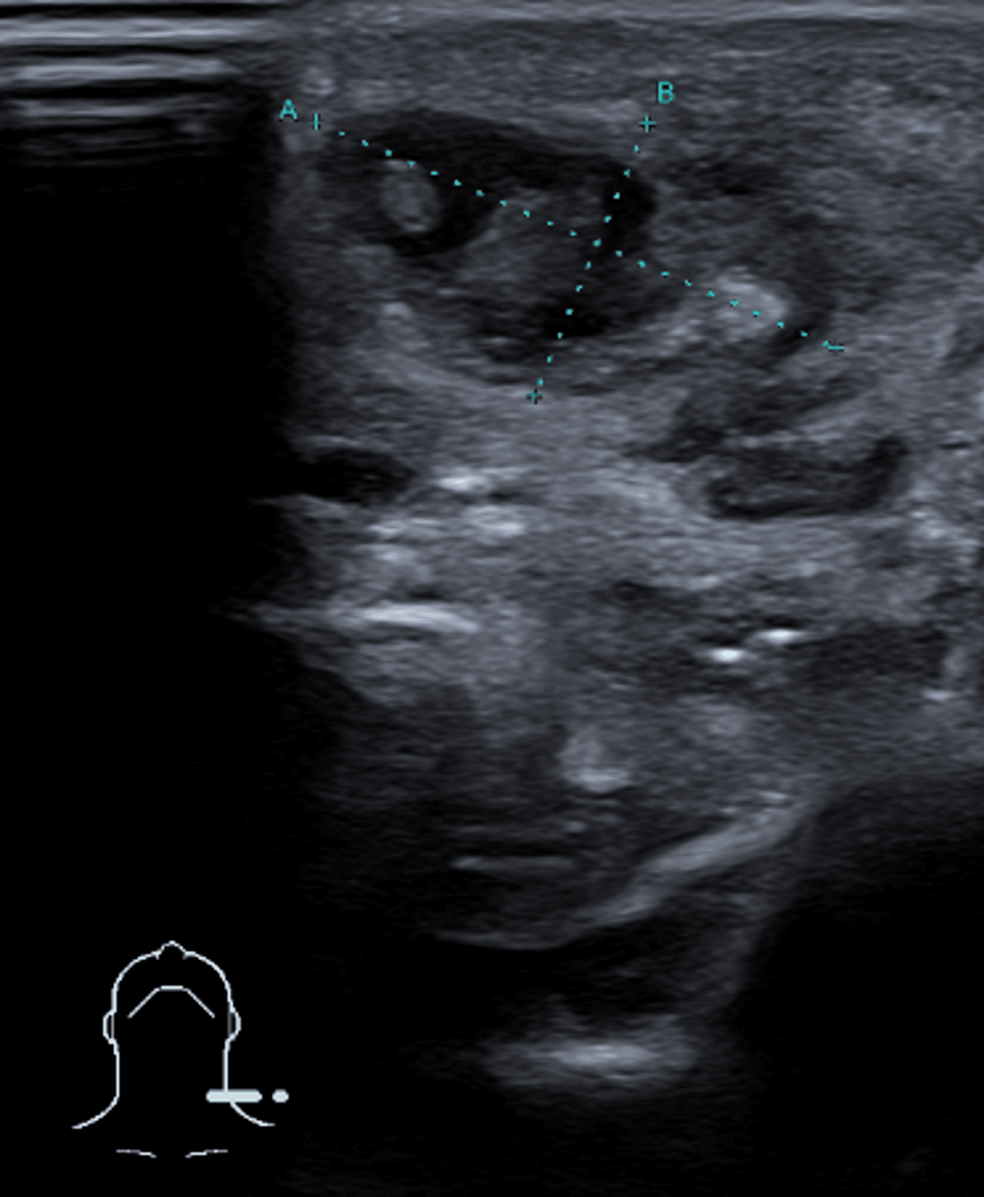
Thyroid ultrasound showing a cervical abscess (20×11×21 mm) in close contact with the left thyroid lobe and isthmus.
